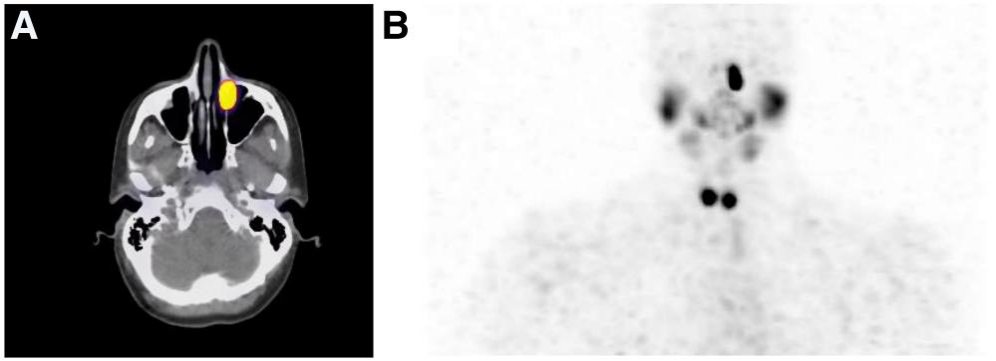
Diagnostic scan with 123-Iodine. A shows the SPECT-CT scan demonstrating increased iodine uptake in the medial portion of the patient's left orbit. B shows the whole-body iodine scan. This scan demonstrates the physiological uptake of the salivary gland and remnant thyroid as well as the irregularity of iodine uptake in the left orbit.
